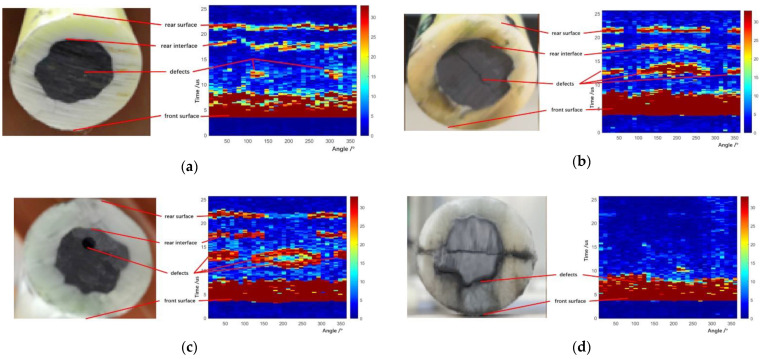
Ultrasonic images of sucker rods with different defects: (a) small crack defect, (b) transverse crack defect, (c) hole defect, and (d) chapped defect.
